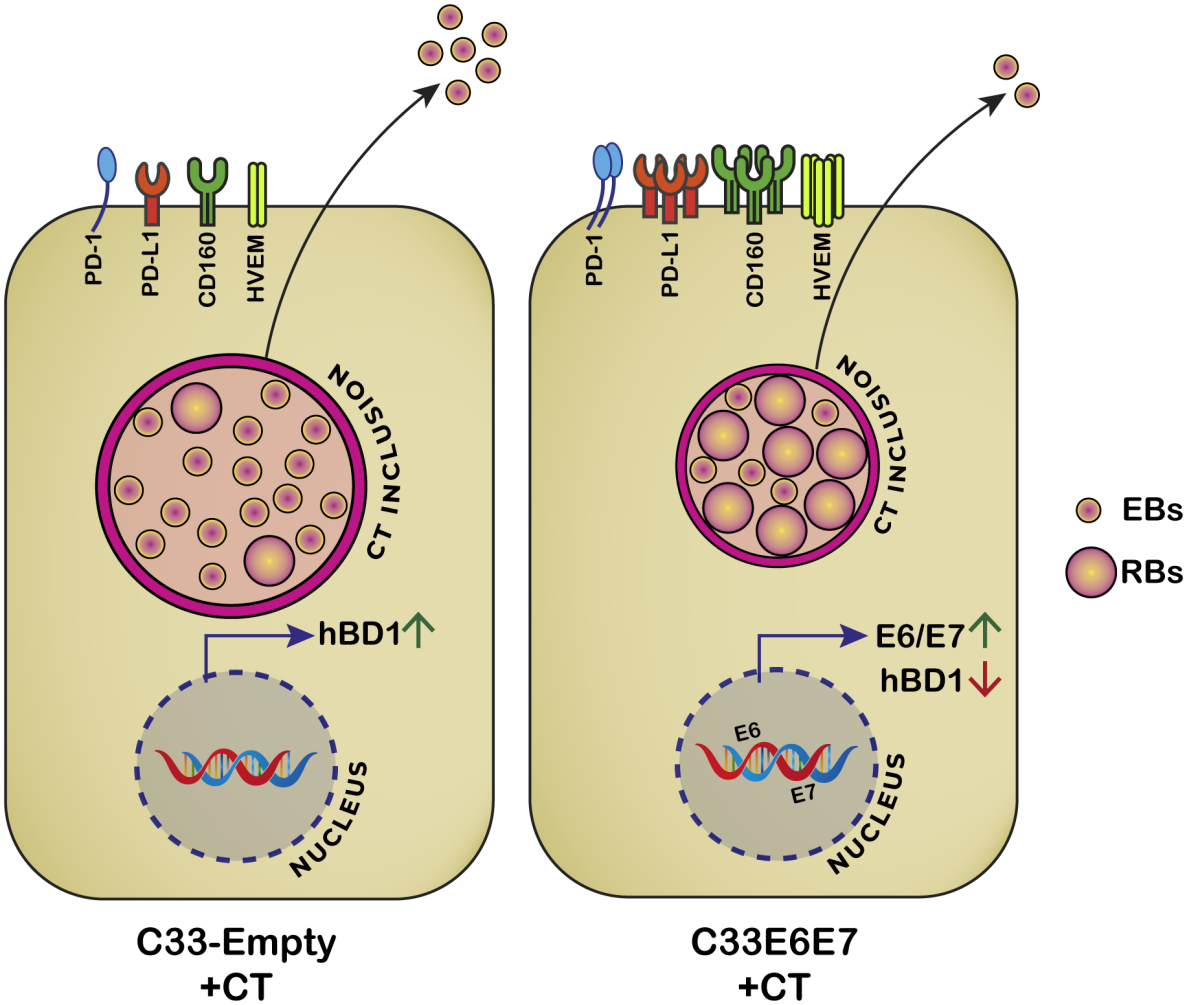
Proposed model for HPV-16 E6 and E7 oncoproteins effects on *C. trachomatis* development and immunoregulatory molecules. When CT infects C33E6E7 cells, smaller inclusions are produced and RB to EB transition is impaired. Consequently, fewer infectious particles are released at the end of the developmental cycle. Simultaneously, increased expression of E6 and E7 oncoproteins and the immunoregulatory molecules PD1, PD-L1, HVEM and CD160 as well as decreased levels of hBD1are observed.
